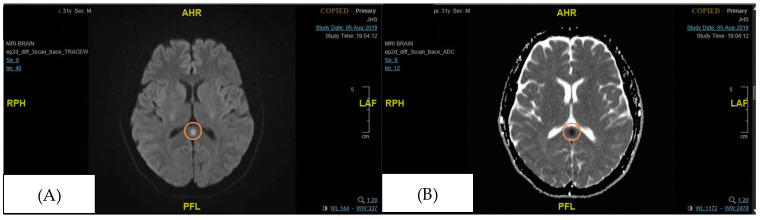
Focal signal abnormality in the splenium of the corpus callosum (initial MRI brain performed during admission). The orange circle signifies the area of the splenium that had high signal intensity. (A) DWI (Diffusion Weighted Imaging), (B) ADC (Apparent Diffusion Coefficient).
